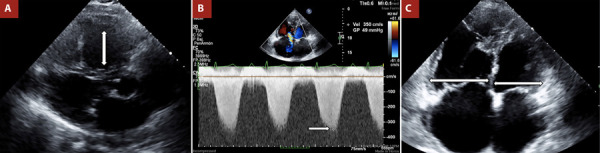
Echocardiographic findings of pulmonary hypertension.
